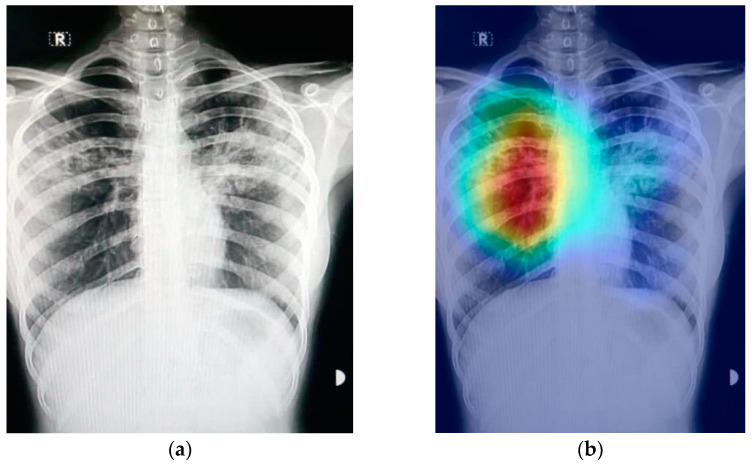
Grad-CAM technique results on the COVID-19 X-ray image: (a) COVID-19 X-ray image; (b) Grad-CAM of the image.
